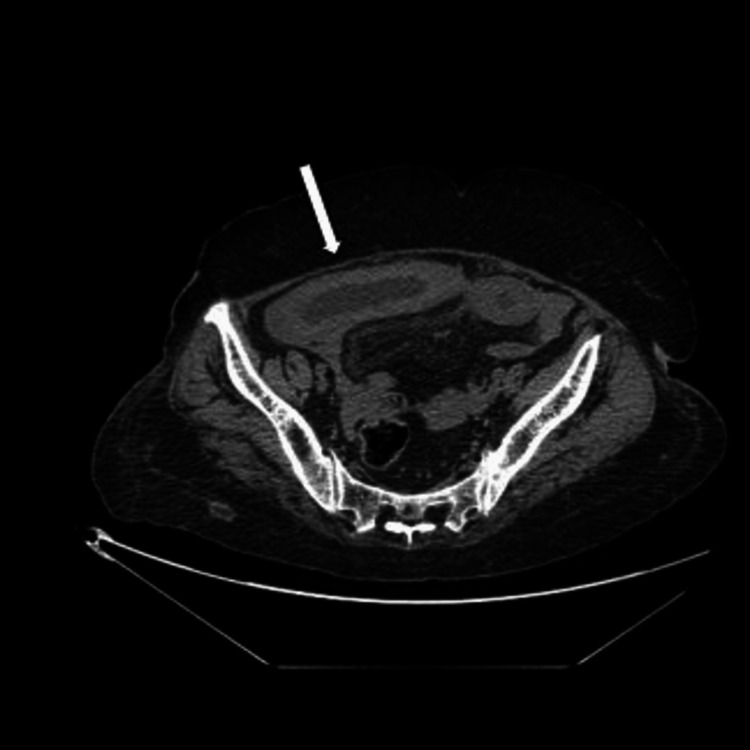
A CT of the abdomen shows circumferential parietal thickening of the grelic gloves.
